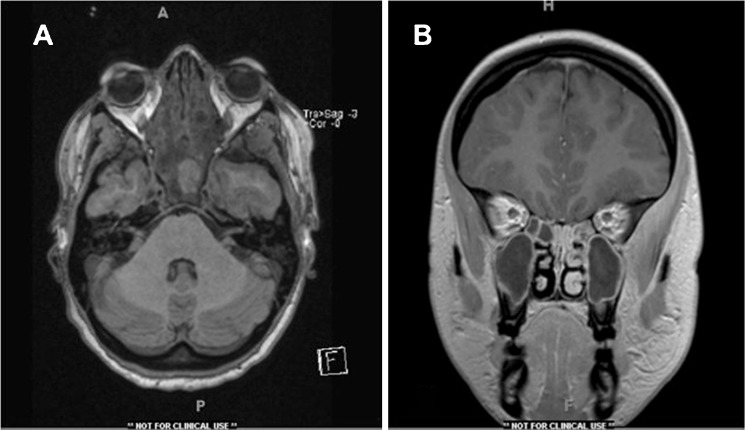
MRI of head and orbits, performed with and without contrast. a Axial view depicting extensive sinusitis. b Coronal view showing thickening within the cavernous sinus with masticator space abscess
